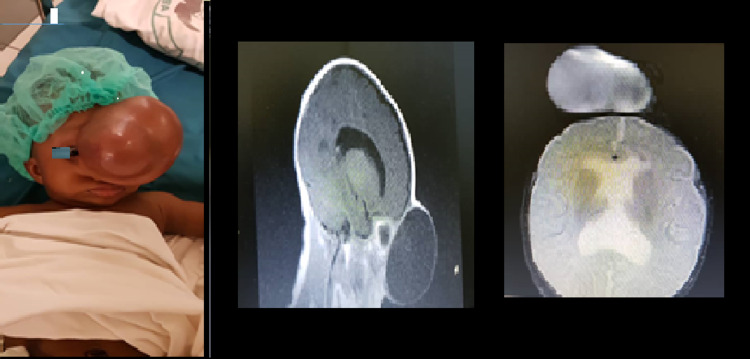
Pre-op evaluation of frontonasal cystic, trans-illuminating mass and MRI showing mostly a cystic frontonasal mass with no brain tissue herniation but with associated hydrocephalus.
